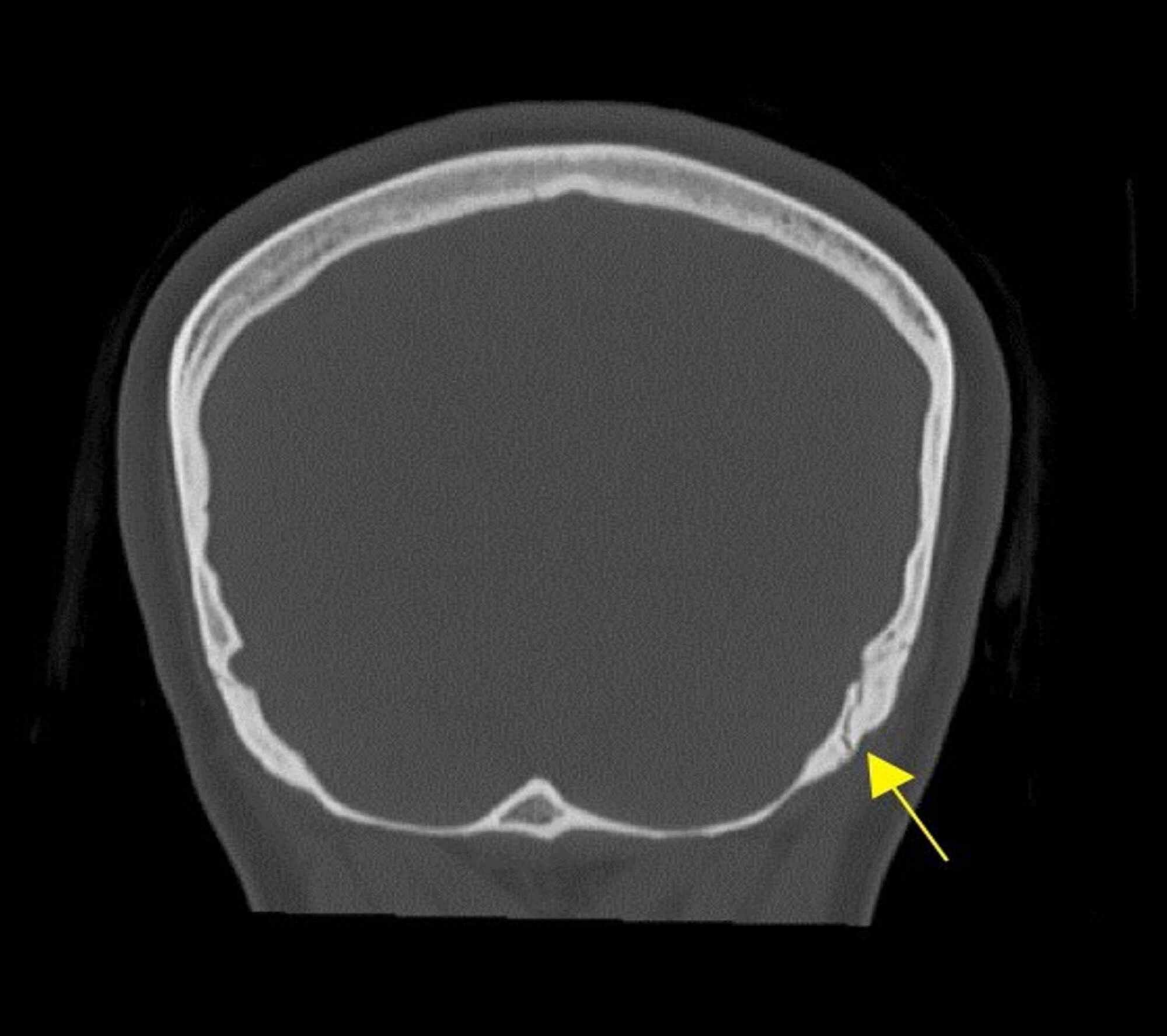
Coronal view of nonenhanced head CT showing the left occipital bone fracture.
